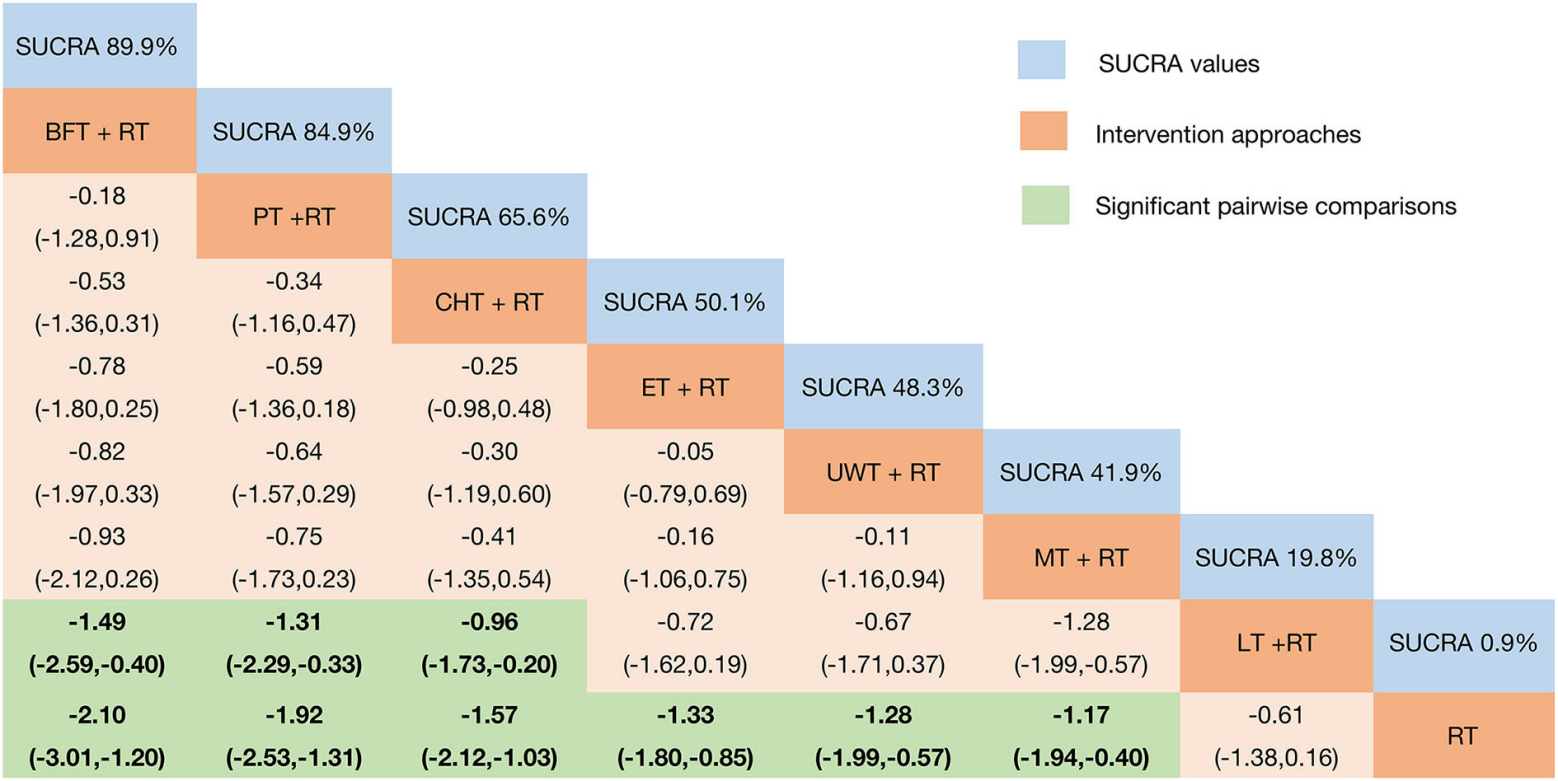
Relative effect sizes of VAS efficacy after the intervention according to network meta-analysis. Treatments were ranked in order of their likelihood of being the best treatment. The numbers in the blue boxes are SUCRA values, representing the rank of the treatments. Meaningful pairwise comparisons are highlighted in green and bold. RT, rehabilitation training; ET, electrotherapy; LT, light therapy; UWT, ultrasonic wave therapy; CHT, conduction heat therapy; PT, pressure therapy; MT, magnetic therapy; BFT, biofeedback therapy.
